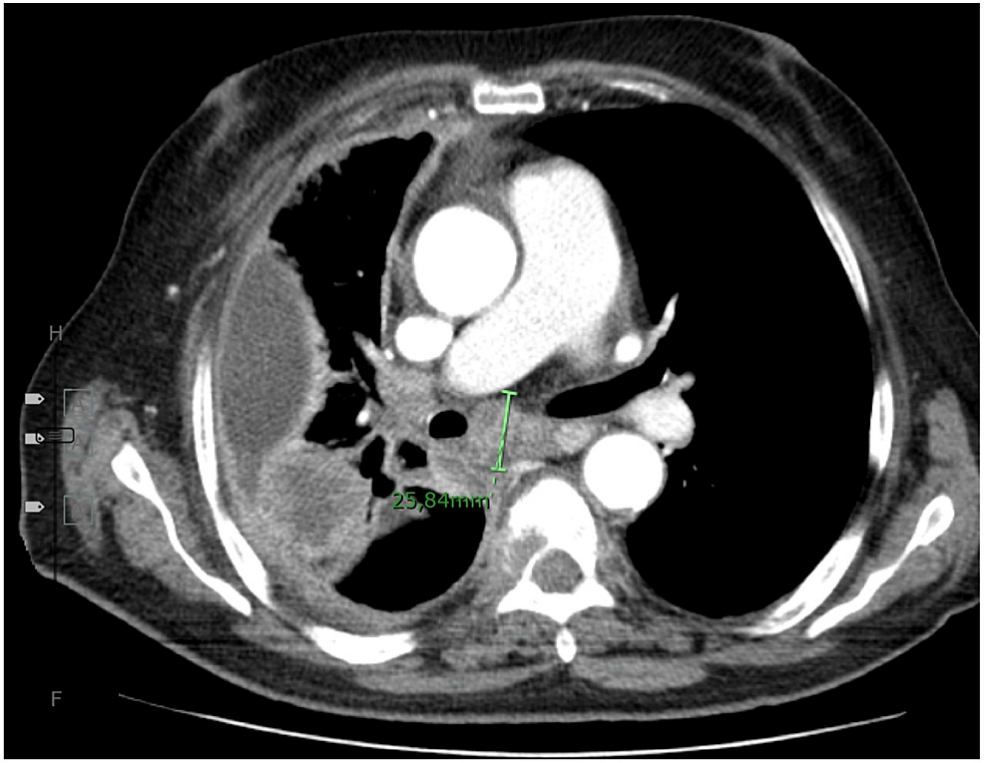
Thoracic section CT scan demonstrated a 2 cm nodular mass and pleural effusion that sugested a bronchogenic neoplastic process.
